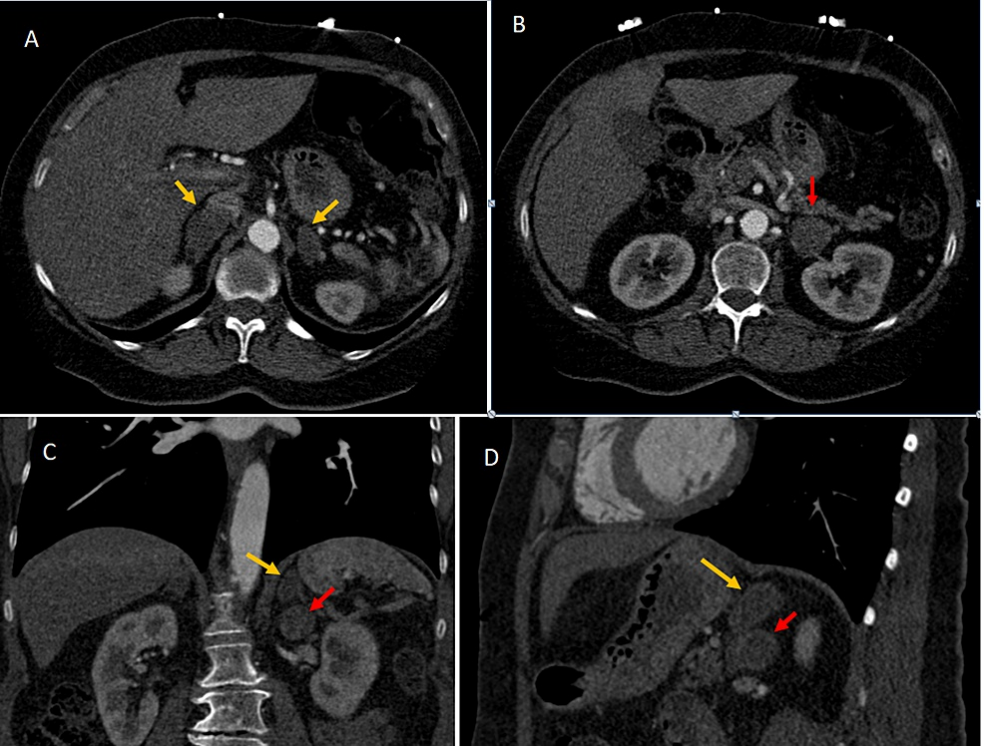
Post-contrast computed tomography (CT) scan abdomen (A) and (B) Axial, (C) Coronal, (D) Sagittal images: Bilateral bulky adrenal glands (orange arrows) with a 2.7 x 3.1 cm rounded lesion in the left renal hilar region (red arrow).
